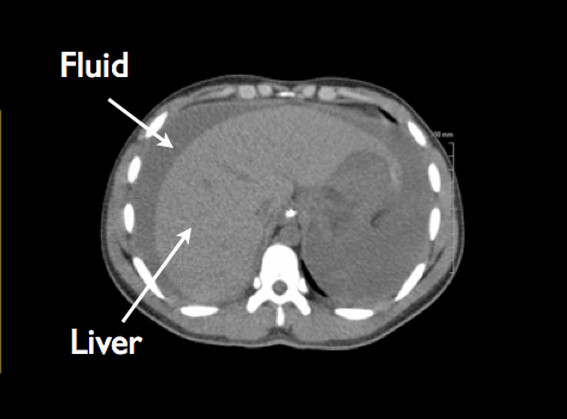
CT of the abdomen showing extensive free fluid.
